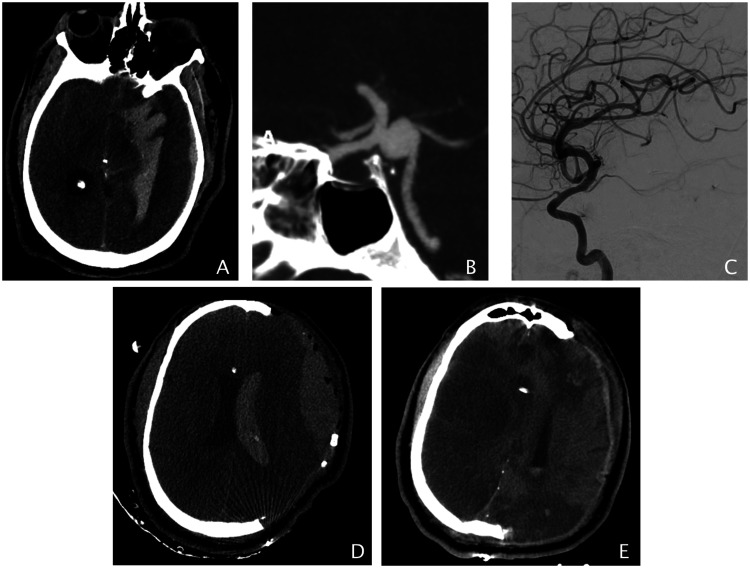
Forty-four-year-old male experienced a sudden loss of consciousness following intense bouts of coughing. (a) and (b) He was diagnosed with ruptured 1.5 cm left PCOM aneurysm causing intraventricular, intracerebral, and subdural hemorrhage. (c) Lateral view of a follow-up DCA post-surgical aneurysm reconstruction and decompressive hemicraniectomy showing complete aneurysm obliteration. (d) Axial view of a non-contrast CT head day 1 post op shows decompression of the brain without a midline shift and a 3 cm epidural hematoma. (e) A follow-up non-contrast CT head four days post op showed increasing brain edema with midline shift, extensive bilateral cerebral infarcts with extension of the infarct into the bilateral occipital.
